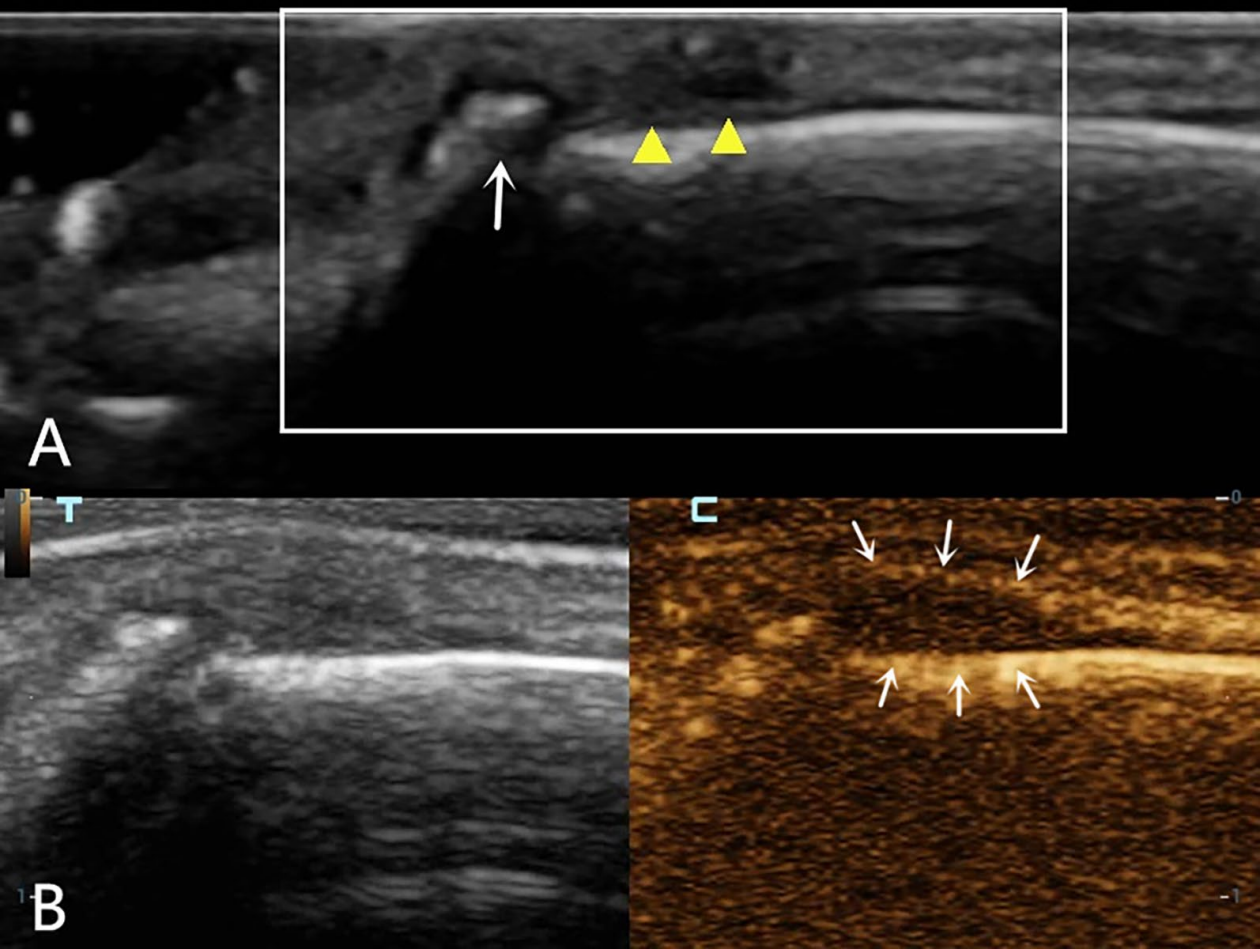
Ultrasound examination of a 34-year-old male with a left fourth finger extensor tendon injury. A. Two-dimensional ultrasound shows thickening and reduced echogenicity of the extensor tendon at the level of the distal interphalangeal joint (indicated by a solid yellow triangle). Avulsion fracture fragments with strong echogenicity can be seen at the base of the distal phalange (white arrow). B. CEUS showing no contrast agent filling inside the extensor tendon (indicated by a white arrow)
